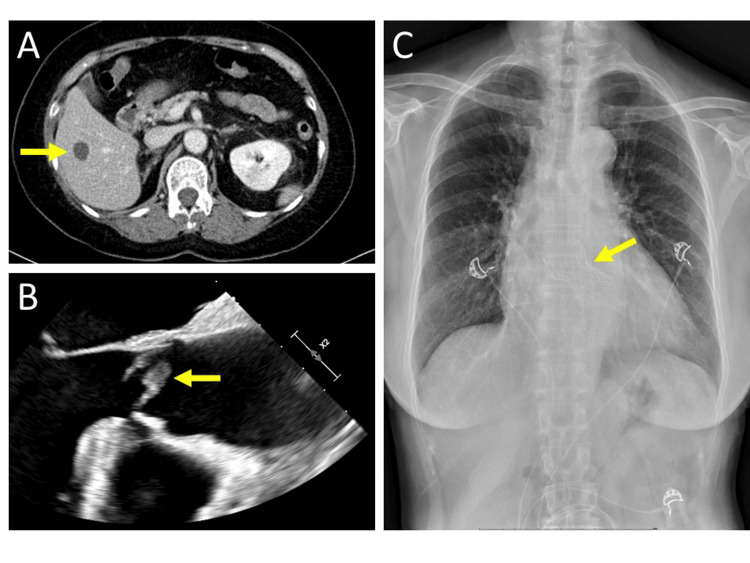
Severe infection characteristics associated with adalimumab and post-treatment follow-up (A) Abdominal contrast-enhanced CT scan showing a hypodense liver lesion (indicated by the yellow arrow), suggestive of a liver abscess. (B) Echocardiography image displaying a bicuspid aortic valve with vegetation attached (indicated by the yellow arrow). (C) Chest X-ray after transcatheter aortic valve replacement (TAVR) demonstrating the presence of a 34 mm CoreValve (indicated by the yellow arrow).
